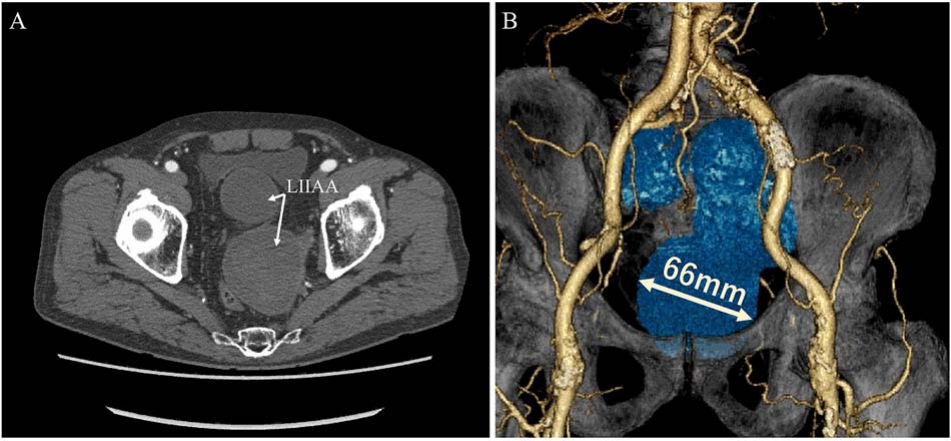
Preoperative computed tomography imaging showing a giant left internal iliac artery aneurysm in a dumpling-like cluster with a maximum short diameter of 66 mm. A—Axial image, B—3D image. LIIAA, left internal iliac artery aneurysm.
